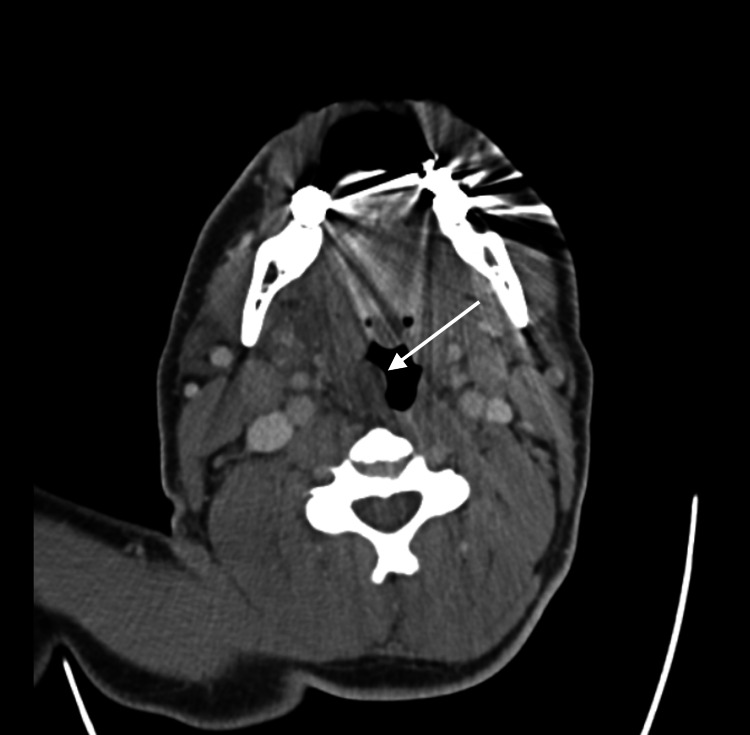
Axial CT of the neck with intravenous contrast demonstrating isolated uvulitis without tonsillitis or other evidence of pharyngitis
